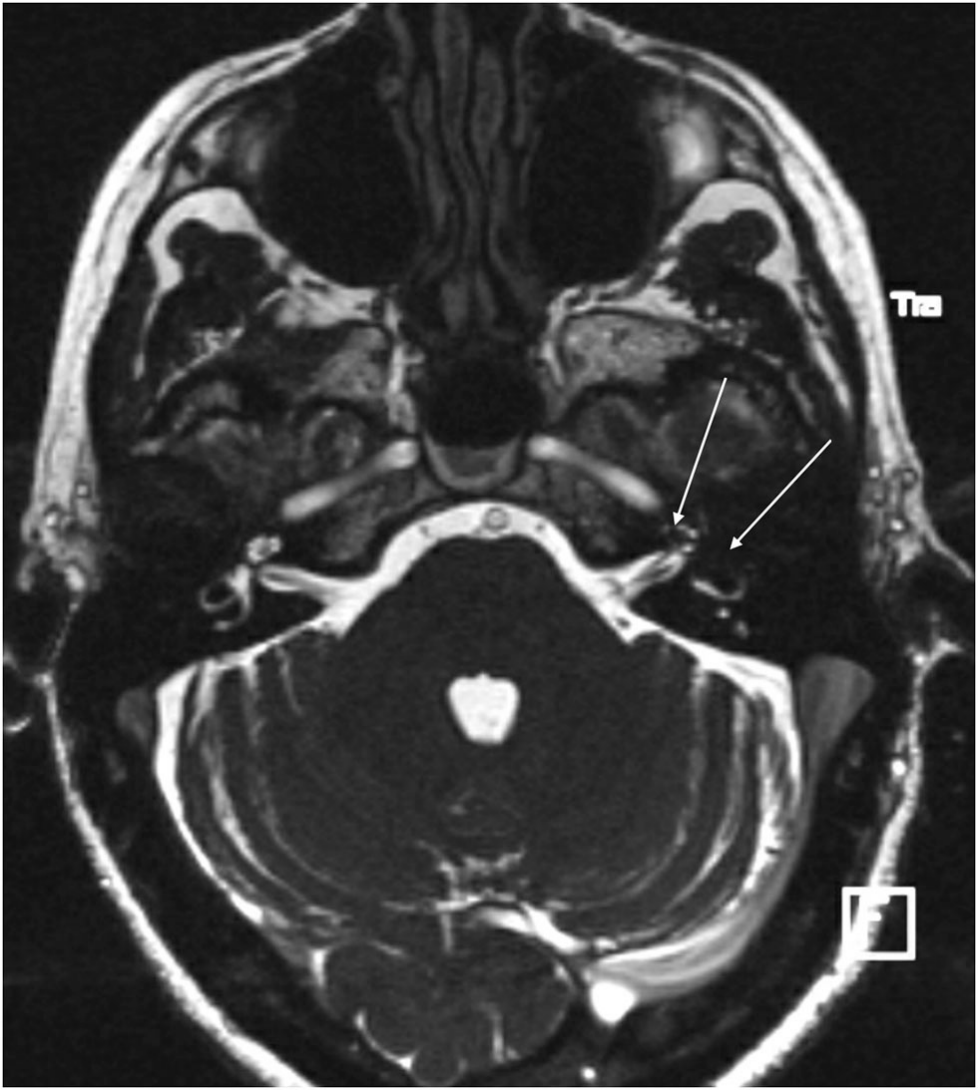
CISS sequence MRI of a patient with PLF showing significant air in the vestibule and the anterior crus of the horizontal canal as well as the second turn of the cochlea (arrows).
